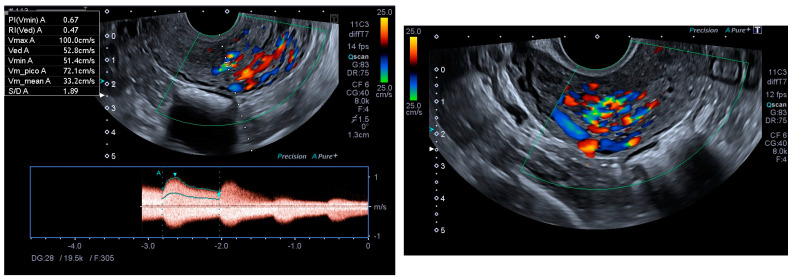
Colour and pulsed Doppler studies of the uterine arteriovenous malformation.
